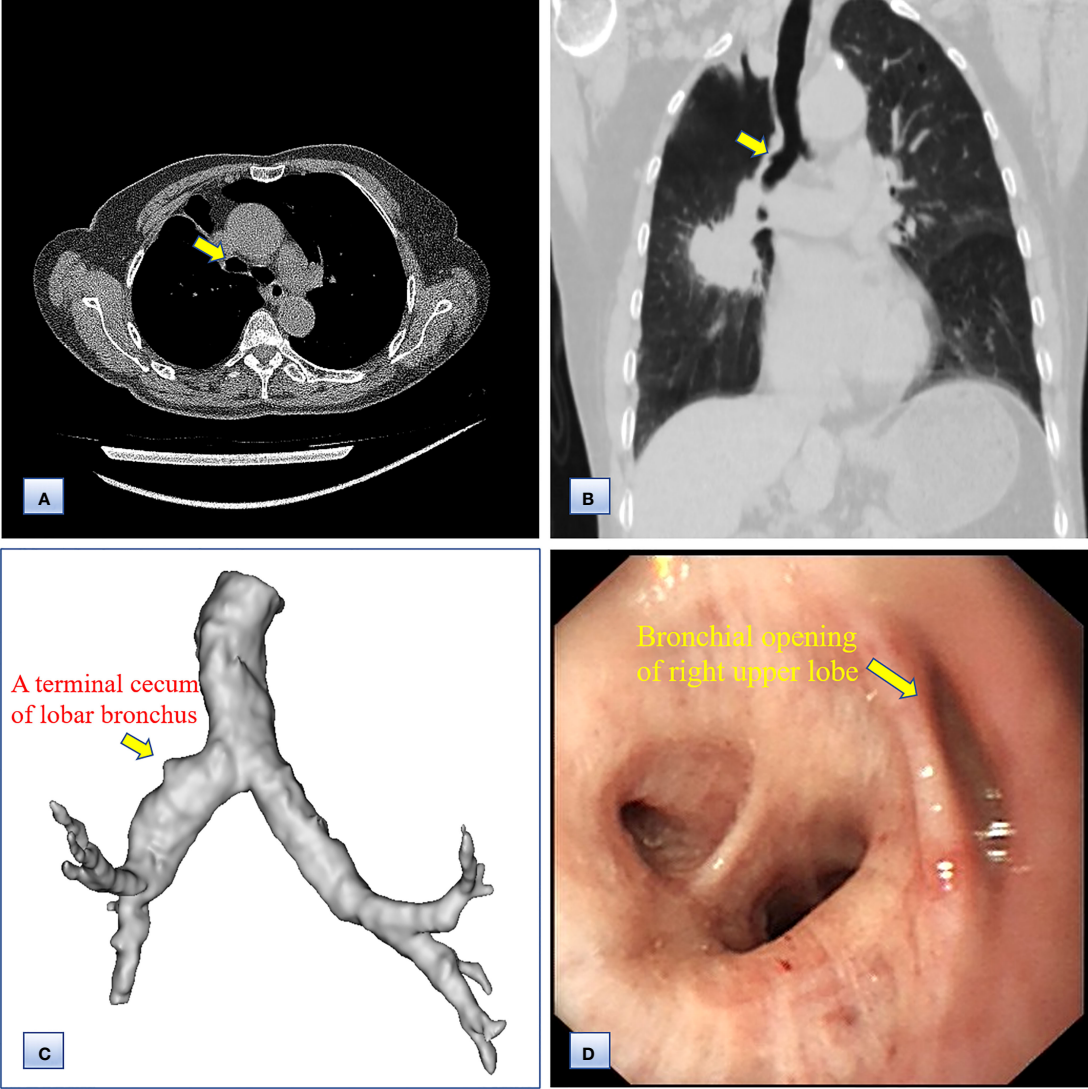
Computed tomography and bronchoscopy examination of the bronchi before surgery. (A, B) Axial (A) and coronal (B) chest CT images, showing the bronchus of the right upper lobe as a bud. (C) 3D CT reconstruction, indicating that the bronchus of the right upper lobe was a terminal cecum, and that the bronchial tree had not formed at the distal end. (D) Bronchoscopy examination, confirming the undeveloped bronchial primordium and ruling out a secondary obstruction.
